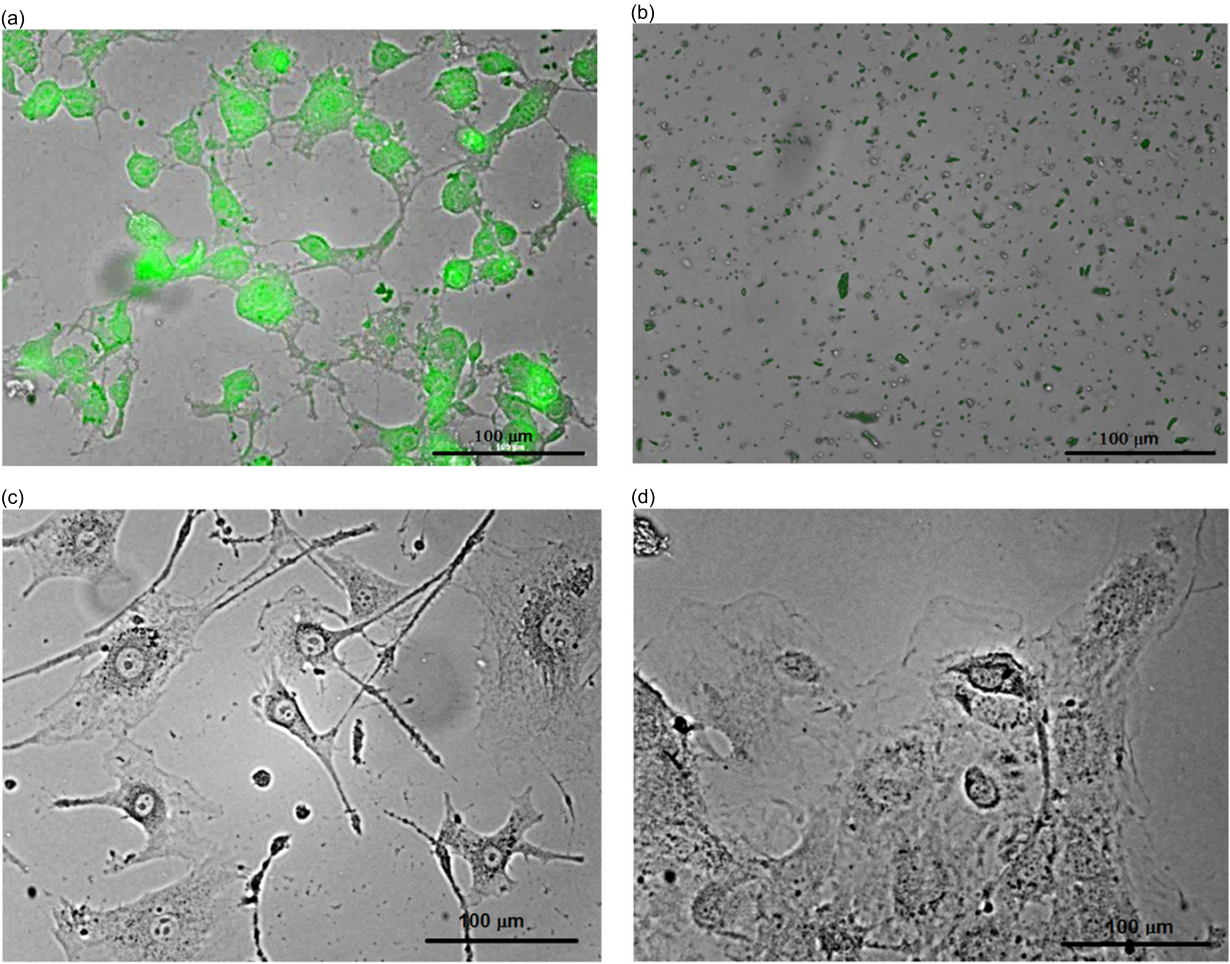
3T3-L1 cells labeled with CFO NPs (1 mg ml^−1^) before (a) and after (b) ultrasound treatment. Non-labeled 3T3-L1 cells before (c) and after (d) ultrasound treatment are shown for comparison.
